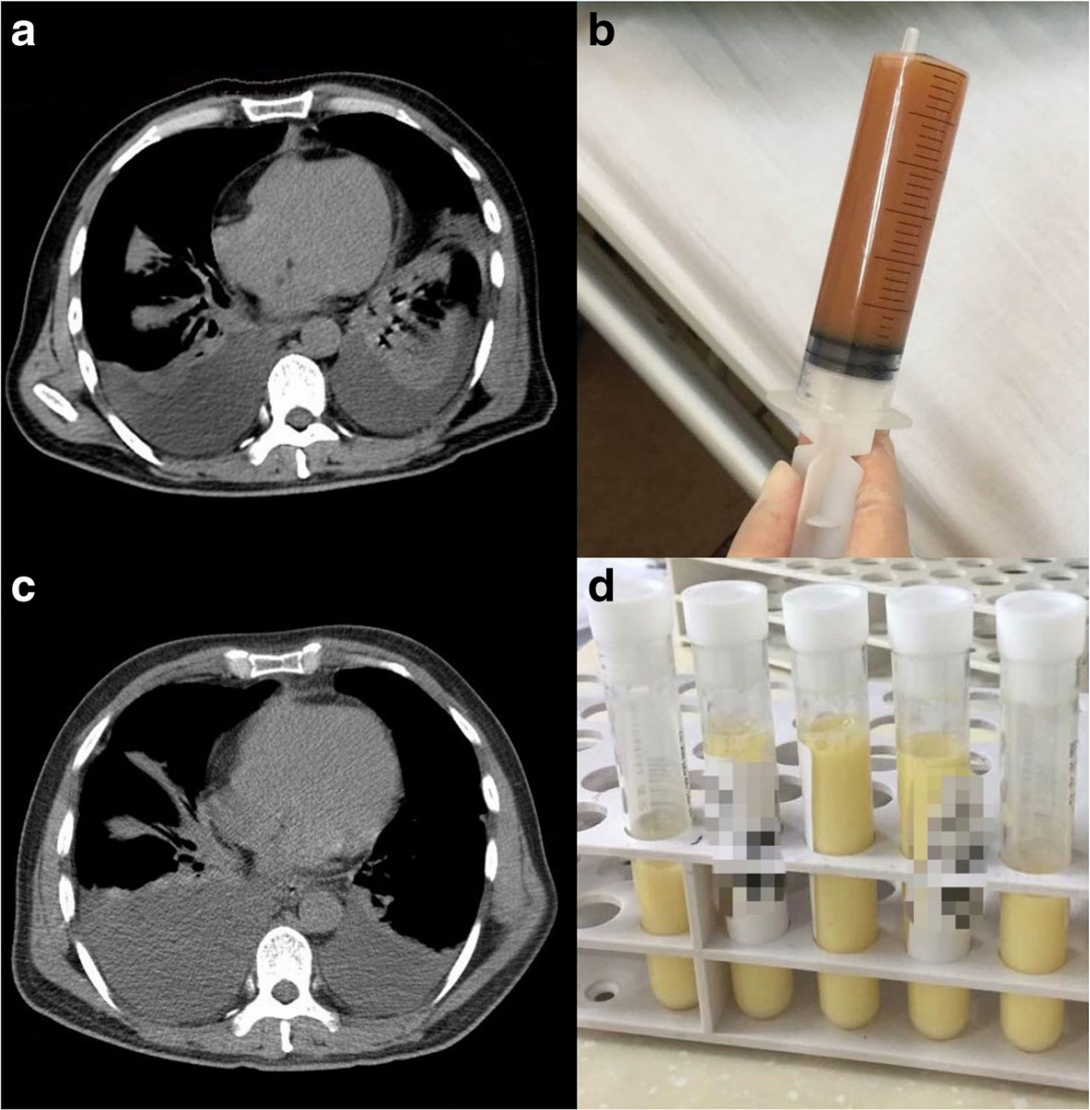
Pleural effusion of a 50-year-old male farmer. a Computed tomography revealed bilateral pleural effusion about a week after the onset of symptom. b Pleural effusion from the first thoracentesis. c Computed tomography showed that pleural effusion continued. d Pleural effusion from the second thoracentesis revealed chylothorax
